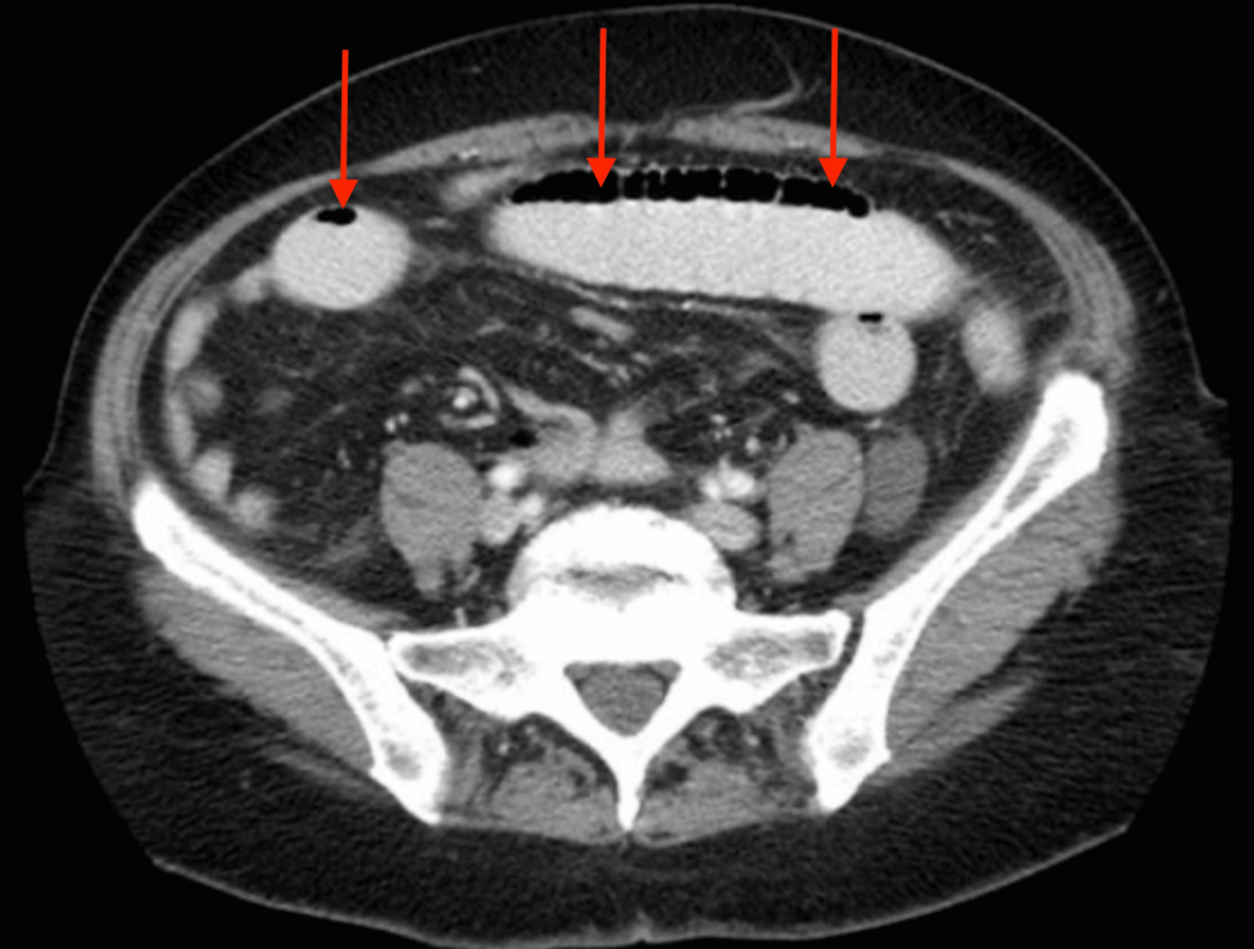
Axial CT scan of the abdomen demonstrating dilated small bowel loops with fluid-filled segments and a paucity of colonic gas, indicative of partial obstruction. Hepatic steatosis and trace ascites are also visible The arrows highlight the dilated bowel segments.
